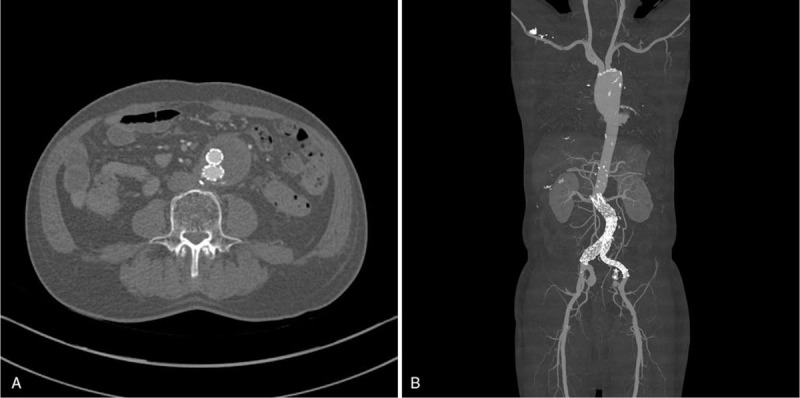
Post-operative CTA shows the complete elimination of type II endoleak. A, Axial view of CTA, B, Reconstruction view of CTA.
